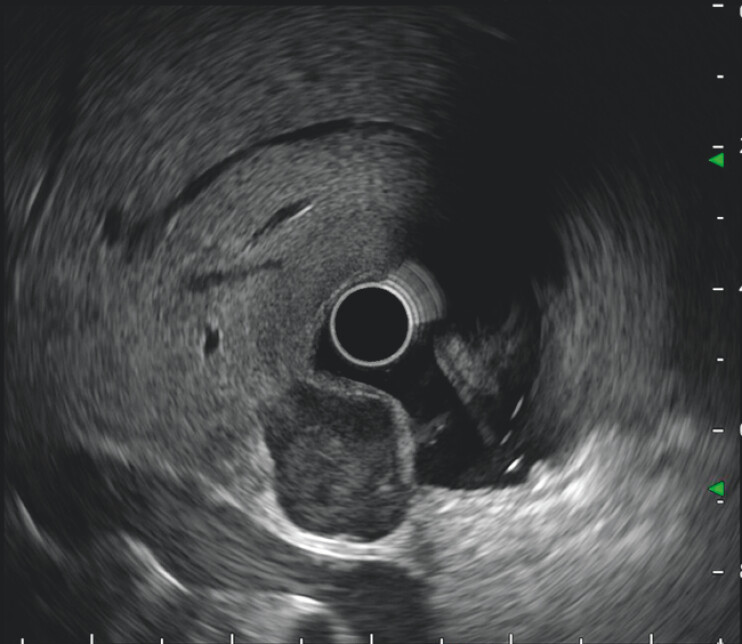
Endoscopic ultrasound showed a heterogeneous hypoechoic lesion originating from the muscularis propria.
